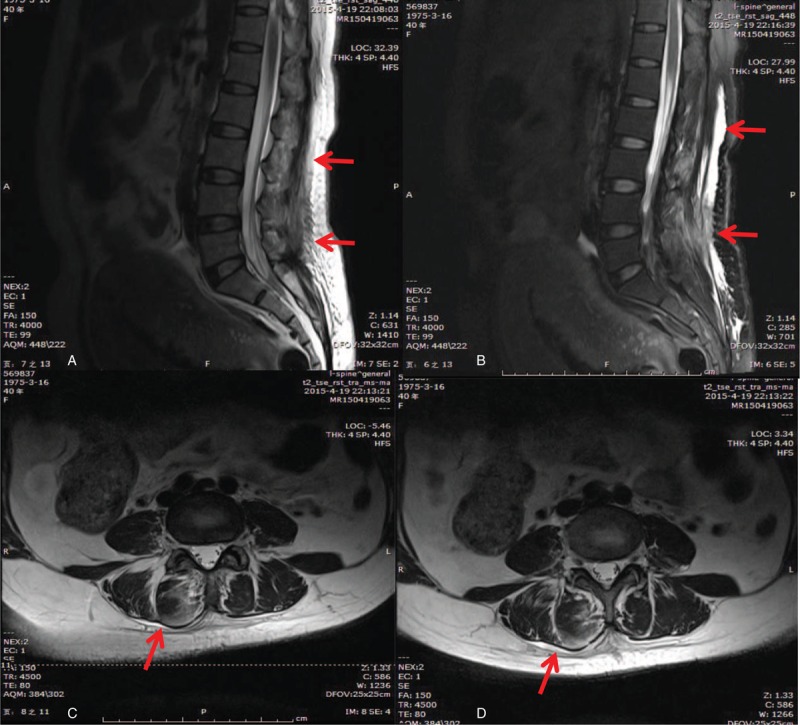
T2-weighted magnetic resonance image scanning without contrast medium on the postpartum Day 2 showed paraspinal muscles infection related to epidural injection. (A) Median sagittal view: the high-intense signal spreads from the subcutaneous tissues to the deep tissues between the L1-L5 interspinal process, indicating a wide and deep muscle involvement. No signal was shown at the epidural space. (B) Median sagittal fat-suppression sequence: a large edematous inflammation/(signal) spreading over subcutaneous tissues (encircled by red arrows). (C) An axial-view T2 image: a large inflammation extending from the superficial subcutaneous tissues to bilateral sides of deep paraspinal muscles (more at the right side) along the epidural injection tract in the L2/3 level. (D) An axial-view T2 image: a large inflammation extending from the superficial subcutaneous tissues to bilateral sides of deep paraspinal muscles (more at the right side) in the L3/4 level.
